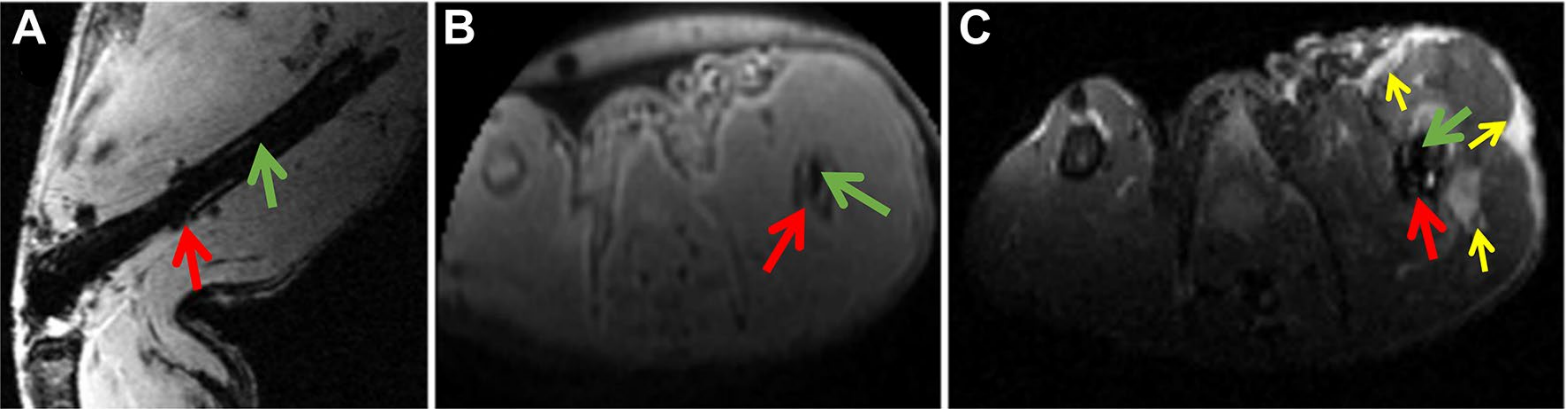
In vivo MRI on day one post fracture showing femur fracture (red arrow), ceramic MouseScrew (green arrow) and edema formation (yellow arrows) in a T2*-weighted (A) UTE (B) and T2-weighted images (C).
